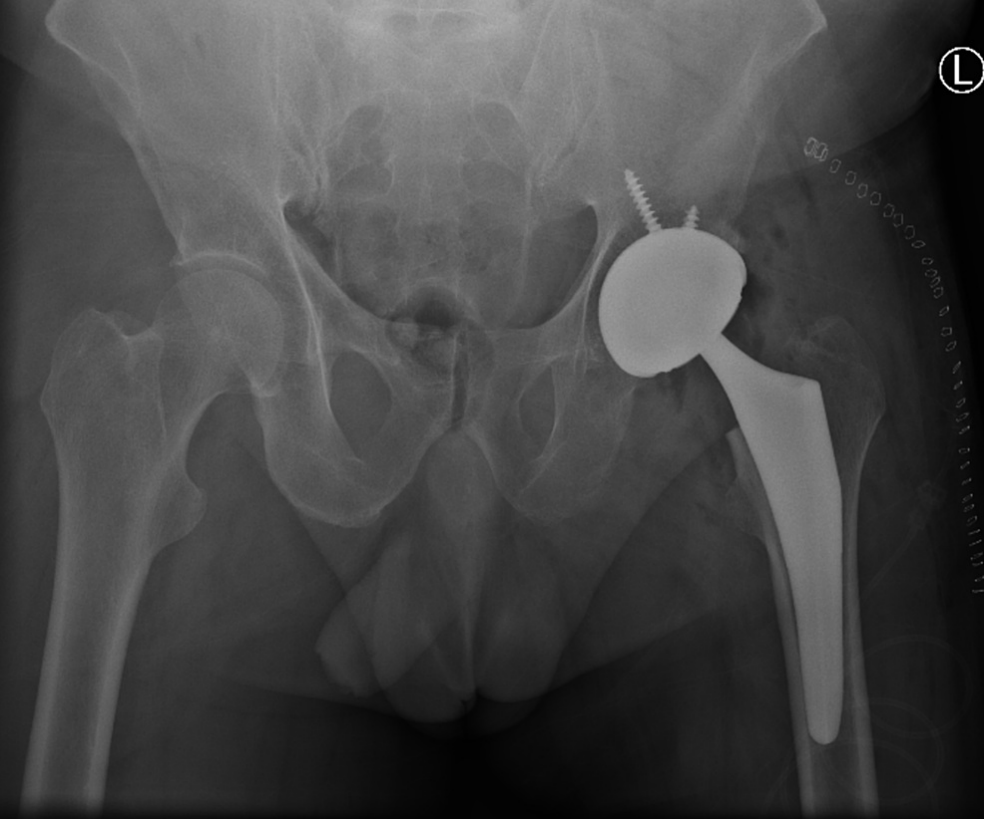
Postoperative anteroposterior radiograph of the pelvis after total hip replacement with a dual mobility system.
